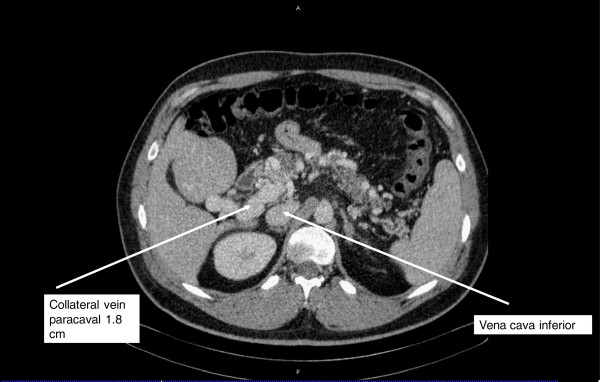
Preoperative computed tomography scan displaying a collateral vein adjacent to the inferior caval vein 1.8 cm in diameter (white arrow) due to portal hypertension caused by extrahepatic portal vein thrombosis.
